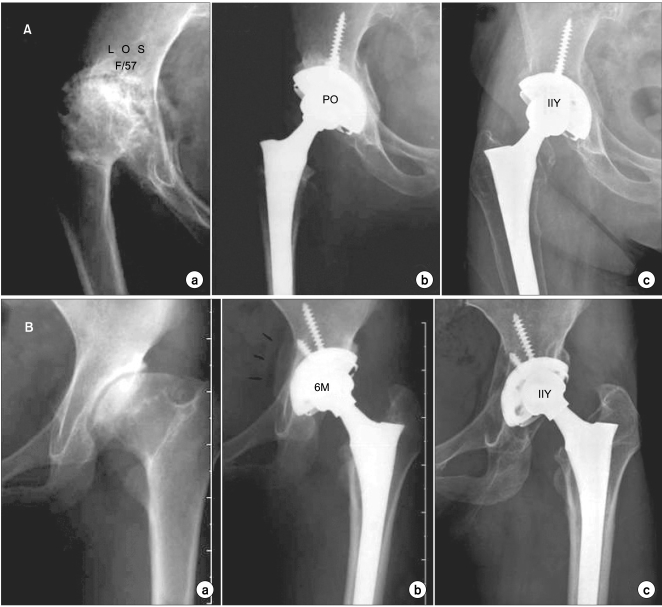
(A) Preoperative (a) and postoperative (b) radiographs in a 57-year-old woman's hip in which a medial wall osteotomy was used in THA to obtain adequate coverage of the acetabular component and a natural center of rotation. (c) Anteroposterior radiograph, taken 11 years postoperatively, showing thick bone stock of the well remodeled medial acetabular wall. (B) Preoperative (a) and 6-month postoperative (b) radiographs in a 21-year-old woman's hip in which a medial wall osteotomy was used in THA to obtain adequate coverage of the acetabular component and a natural center of rotation. (c) Anteroposterior radiograph, taken 11 years postoperatively, showing thick bone stock of the well remodeled medial acetabular wall.
